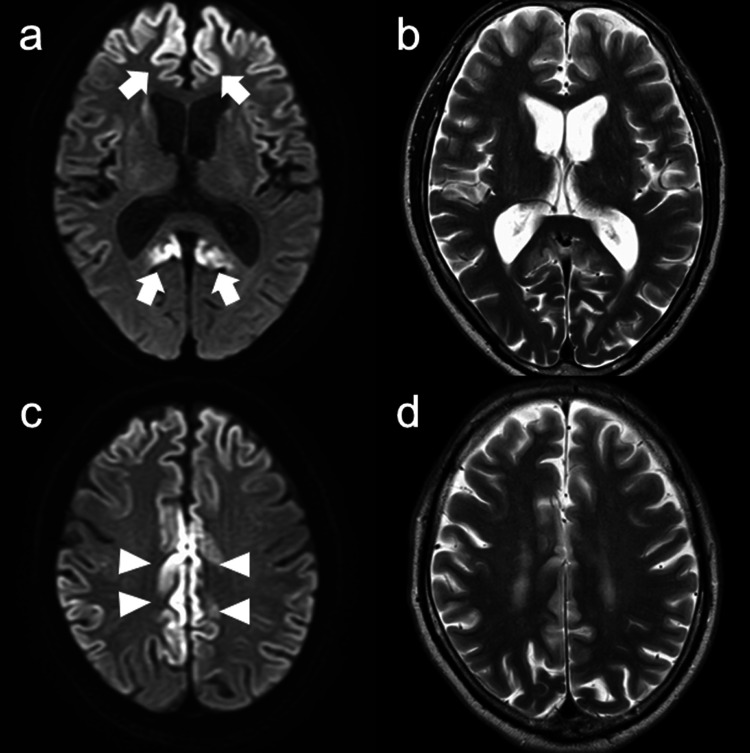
Brain magnetic resonance imaging (MRI) findings at diagnosis. Brain MRI reveals extension of abnormal signals along the cerebral cortices with swelling on diffusion-weighted images (A, C) and T2-weighted images (B, D).
